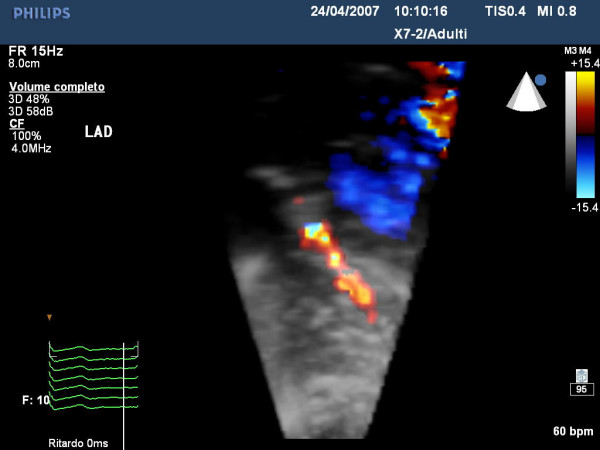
shows an example of the distal tract of left descending coronary artery highlights by 3-Dimensional Color Doppler evaluation.
